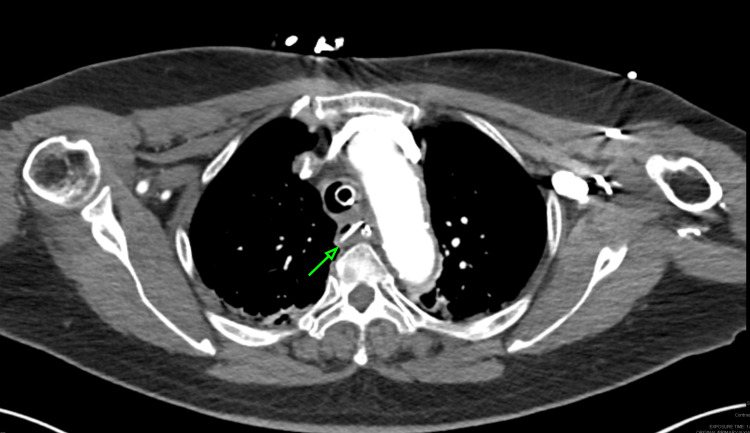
CT of the chest showing a well-positioned endotracheal tube within the trachea, along with a fishbone foreign body in the mid-esophagus. The green arrow identifies the fishbone CT: computed tomography
